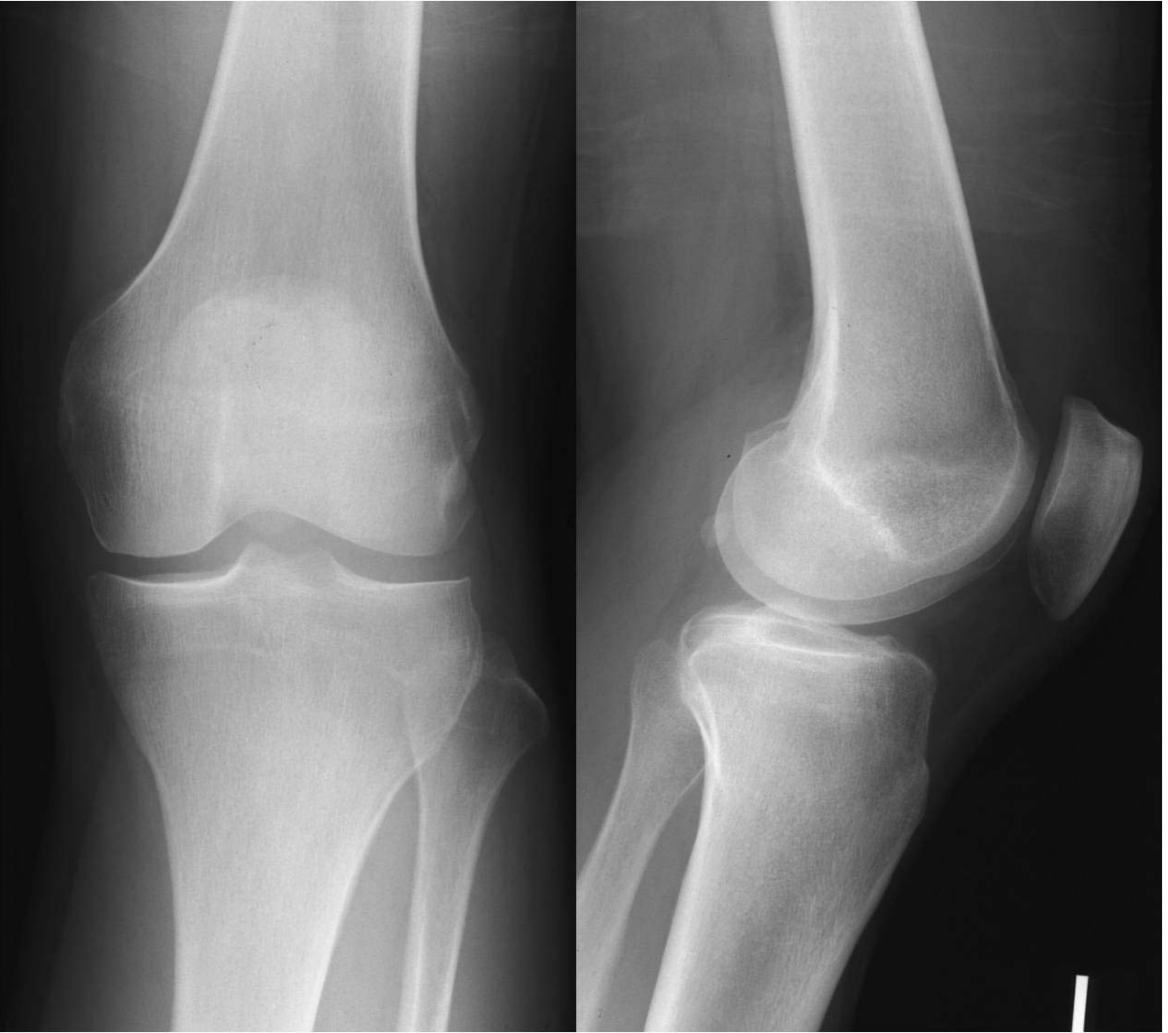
Plain radiograph of the left knee at the time of initialvisit.
